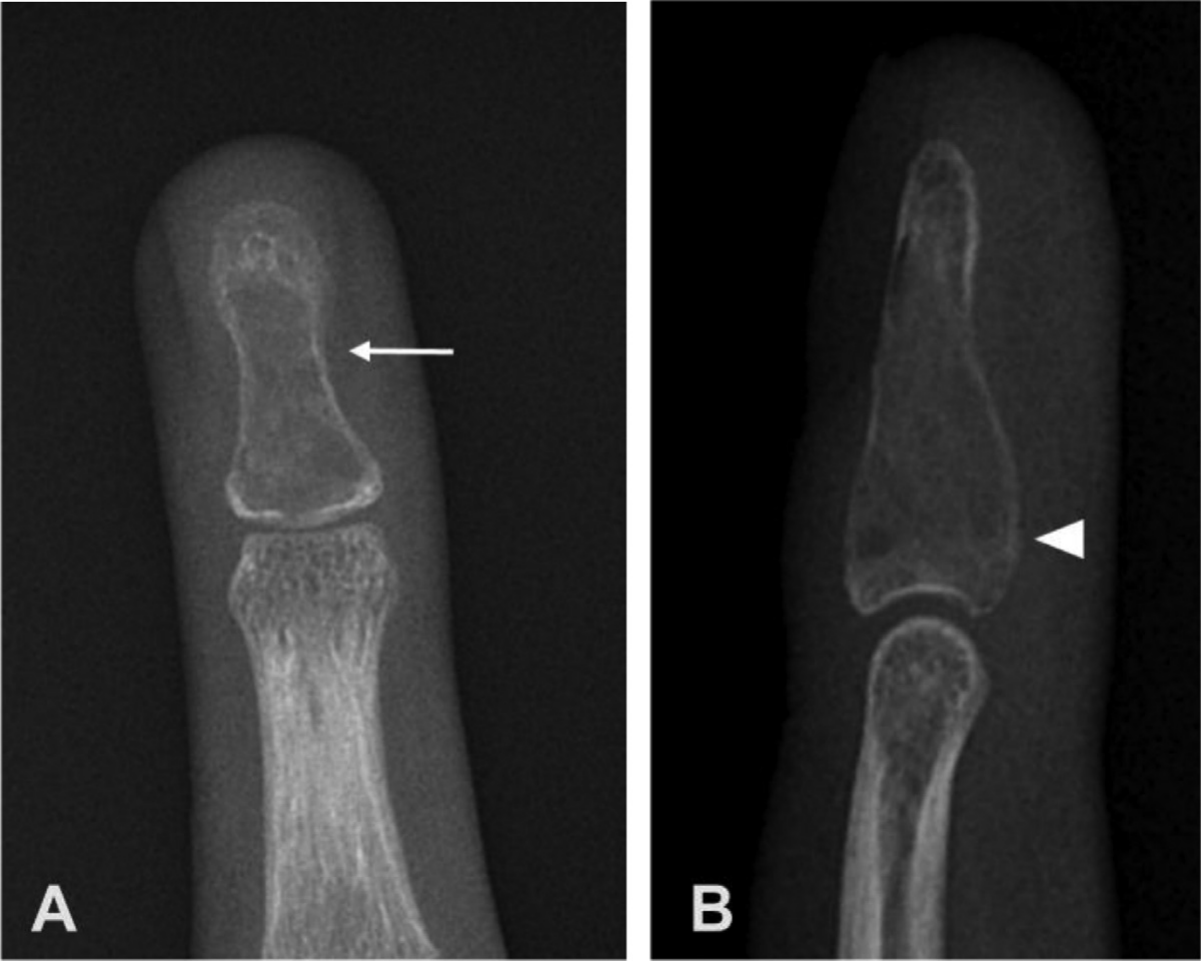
(A) PA and (B) lateral radiograph of the left hand showing a focal lytic, expansile lesion (white arrow) in fourth distal phalanx with minimal adjacent periostitis (arrowhead) and minimal fourth digit soft tissue swelling.
